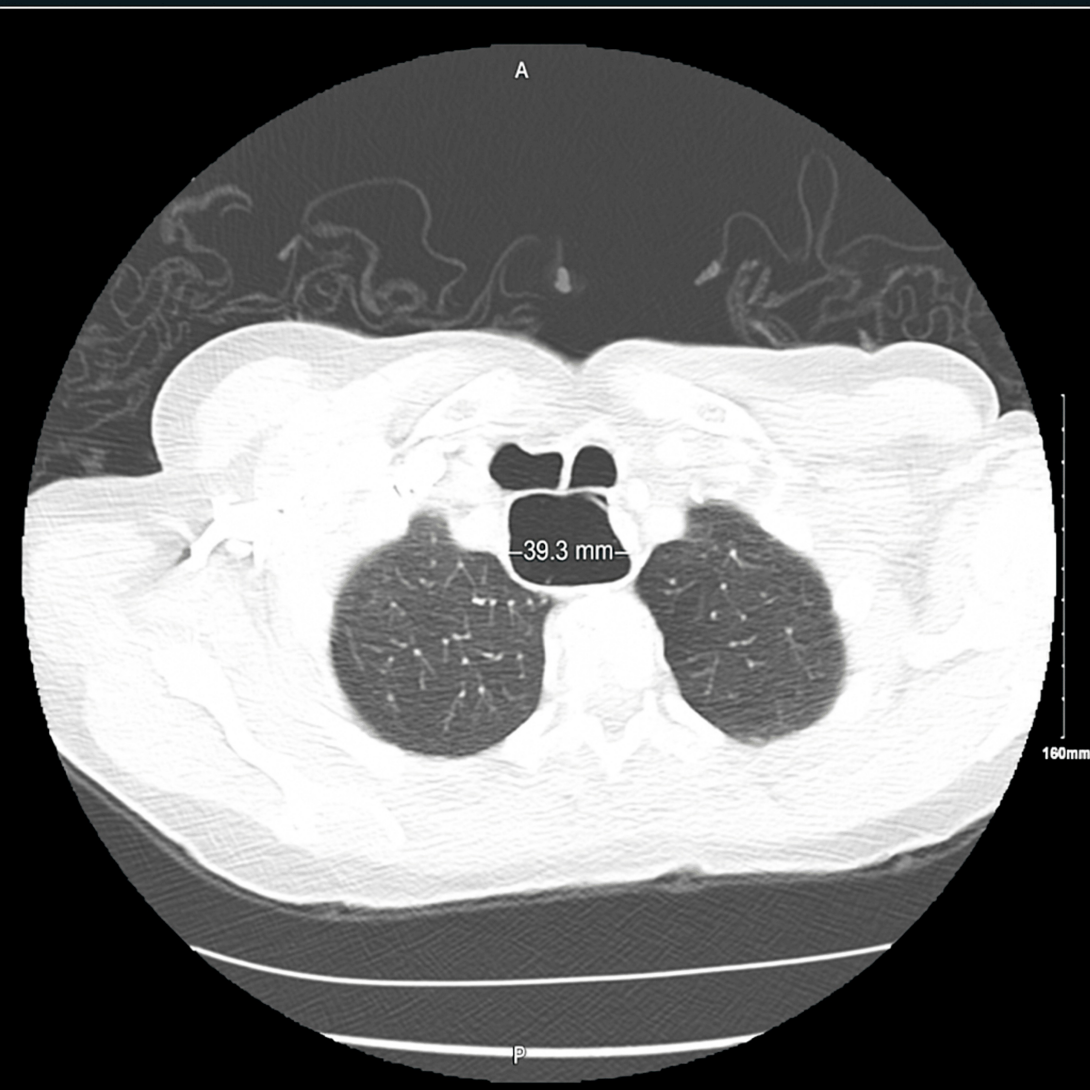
CT scan displaying a 39.3 mm tracheocele along the right lateral tracheal wall, indicative of significant airway dilatation.
